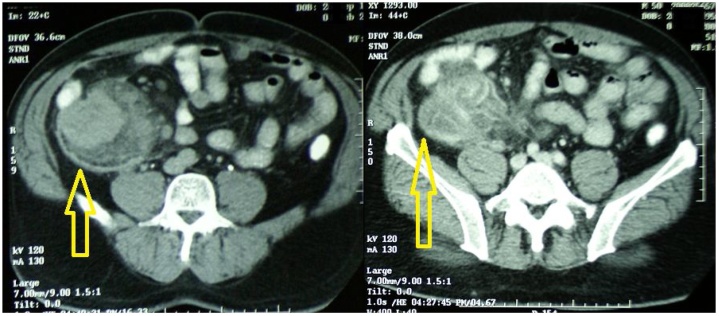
CT scan of the abdomen: (arrows) shows the pseudotumor.
